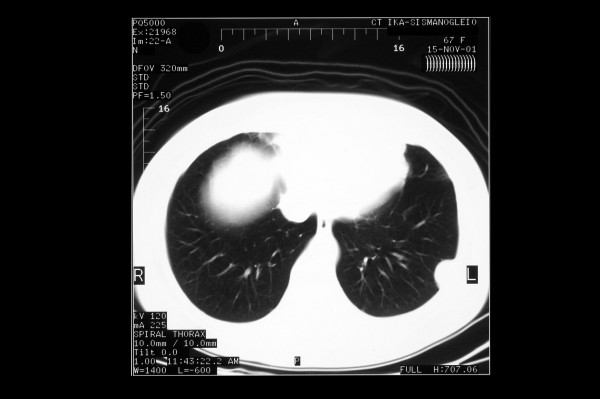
Chest computed tomography (CT).
